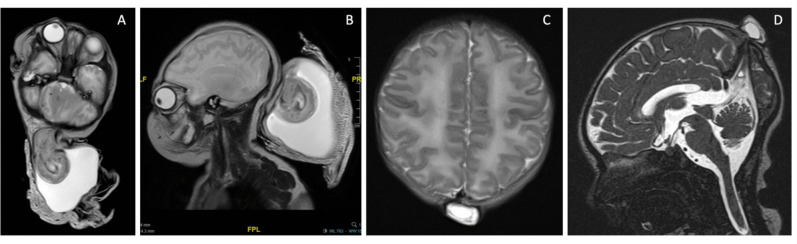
A T2-weighted MRI of an occipital encephalocele with herniation of cerebral and meningeal tissue, axial view (A); sagittal view (B); C–D T2-weighted MRI of a parietal meningocele characterized by the presence of meningeal tissue, liquid content and absence of cerebral tissue, axial view C), sagittal view (D)
